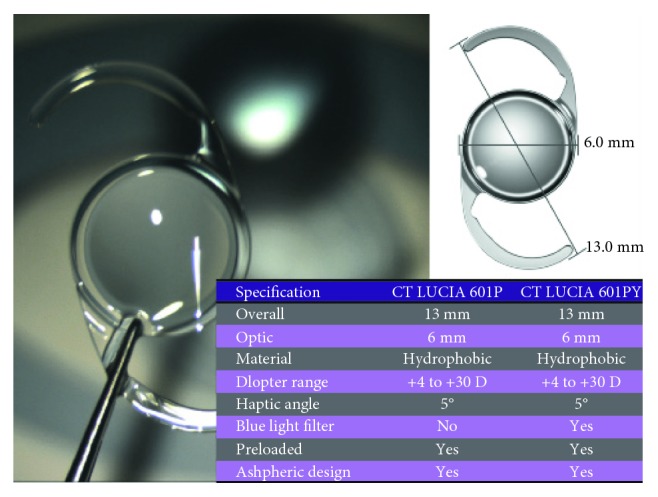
Specifications of the acrylic, hydrophobic CT LUCIA 601(P) (Zeiss, Germany).
